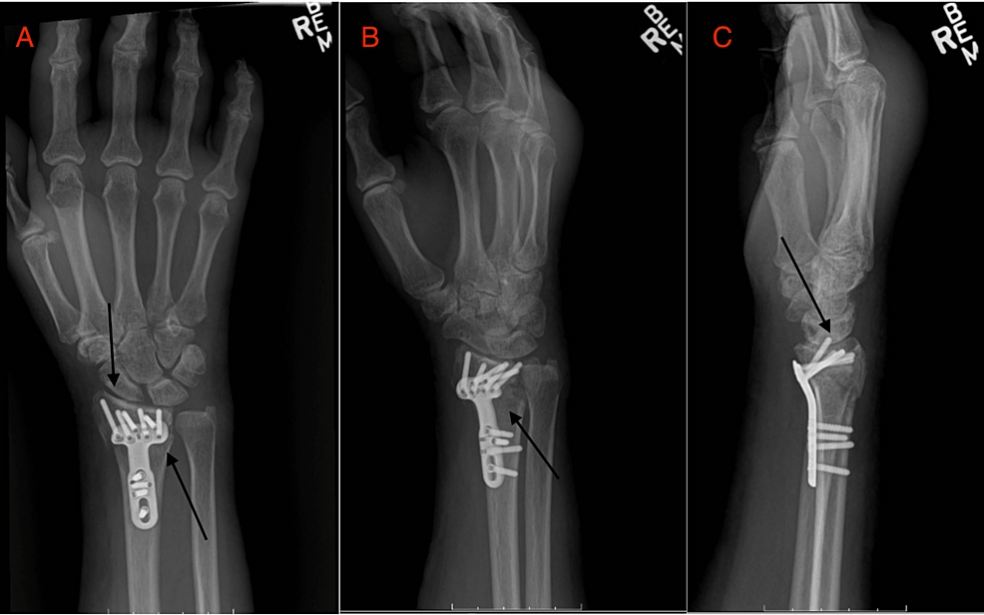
Posteroanterior (A), oblique (B), and lateral (C) radiographs of the right wrist taken postoperatively. A volar locking plate is in place, and anatomic parameters such as volar tilt, radial inclination, and radial height have been restored.
